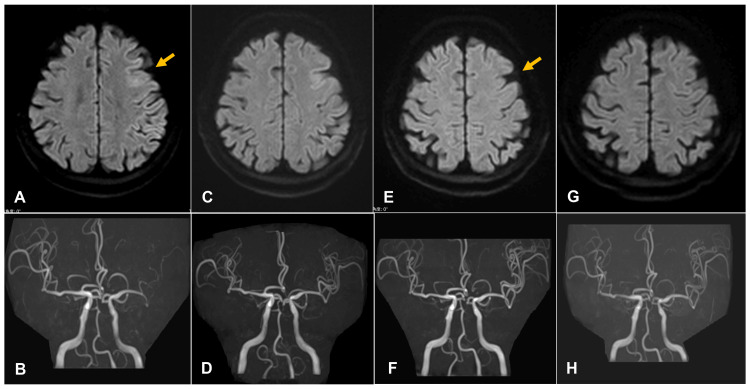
Changes in the image findings CAS, carotid artery stenting; DWI, diffusion-weighted imaging; FSD, fasudil hydrochloride; MCA, middle cerebral artery; MRI, magnetic resonance imaging. (A,B) MRI obtained the day after CAS, when symptoms worsened, shows a small high-signal area on DWI in the left frontal cortex (yellow arrow), with decreased vessel visualization. (C,D) MRI performed the day after local intra-arterial infusion of FSD shows improved visualization of the MCA and resolution of vasospasm. (E,F) MRI taken one week after CAS shows improvement in the abnormal signal (yellow arrow), with slightly increased MCA prominence. (G,H) Follow-up MRI shows normalization of findings.
